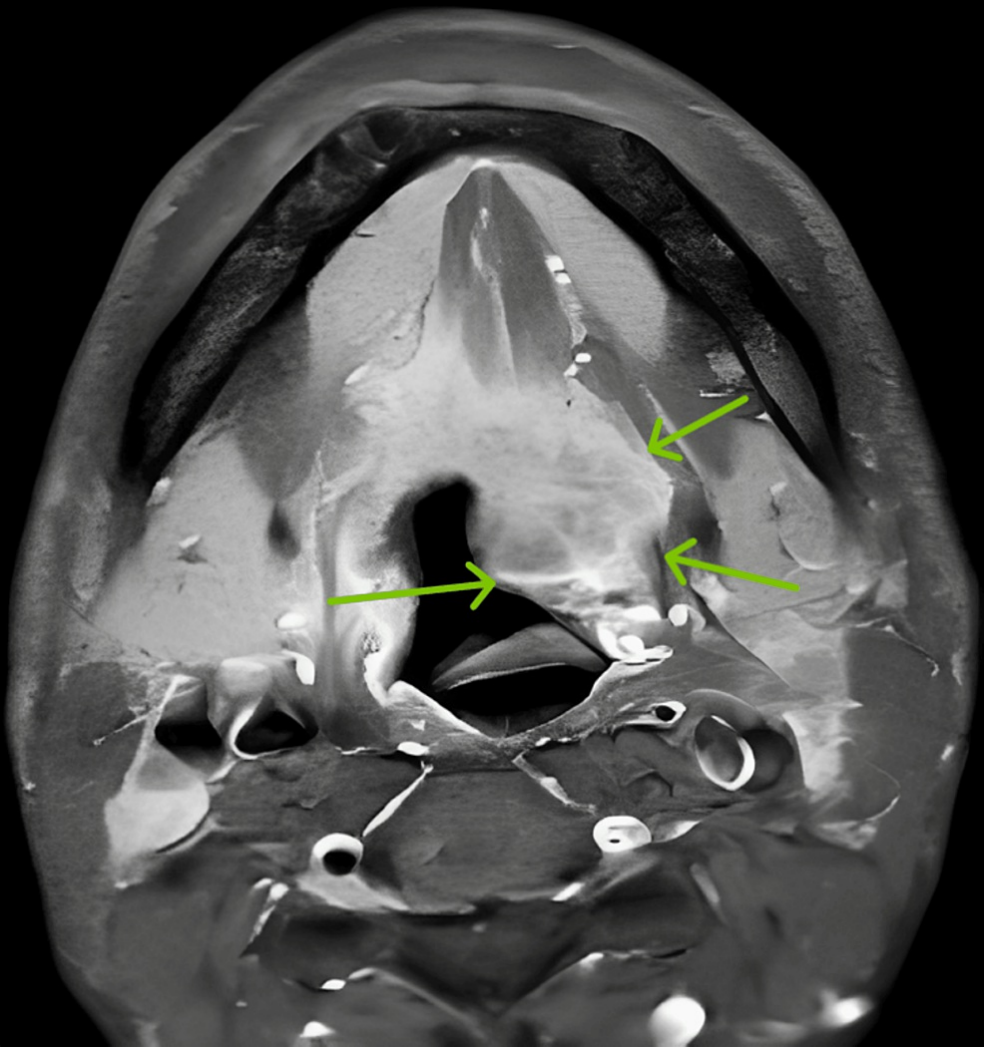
Contrast-enhanced MRI tongue with neck plain with contrast study shows the axial section of a post-contrast T1 fat saturation (FS) image showing a heterogeneously enhancing soft tissue intensity lesion involving bilateral genioglossus with a subtle post-contrast enhancement also seen in the supraglottic region (shown by green arrows)
